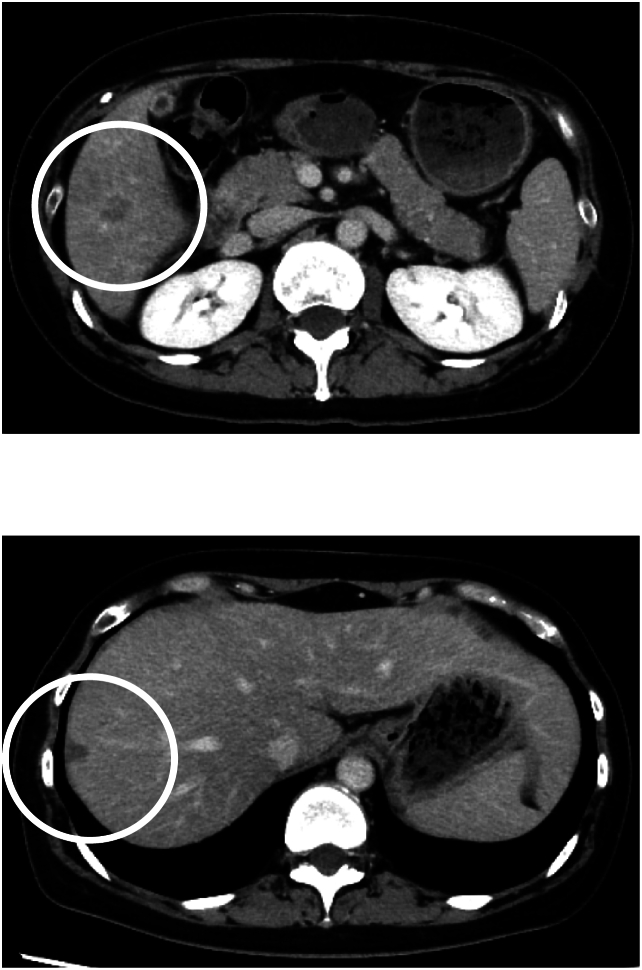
CT scan identifying two liver metastases and 11 disappearing liver metastases (white circles).
